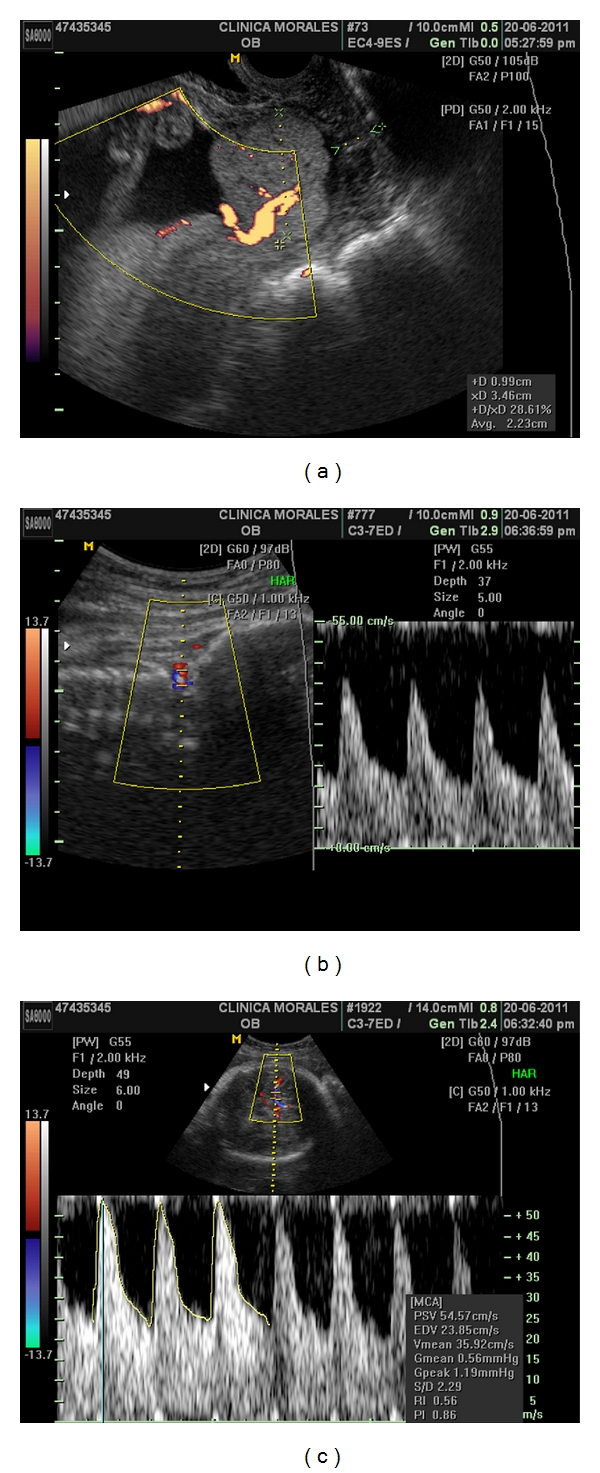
Week 20. Transvaginal colour Doppler ultrasound. The aberrant cotyledon is clearly seen covering the internal os and seems to include the cord insertion. The cervix is very short (9.9 mm) (a) and the Dopper impedances of both the VA and MCA are very low (RI 0.55/PI 0,86) (b, c).
